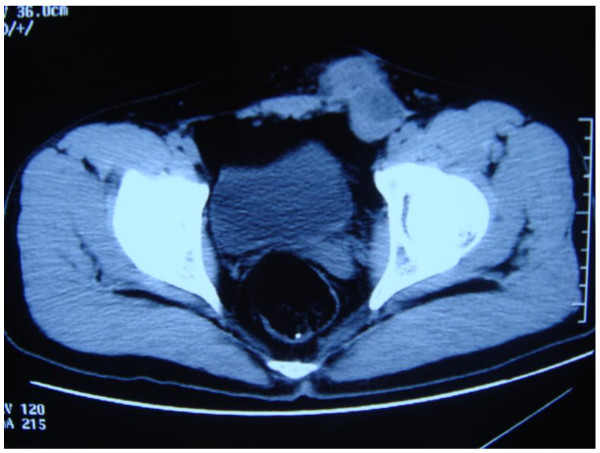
Left inguinal mass on the CT scan.
